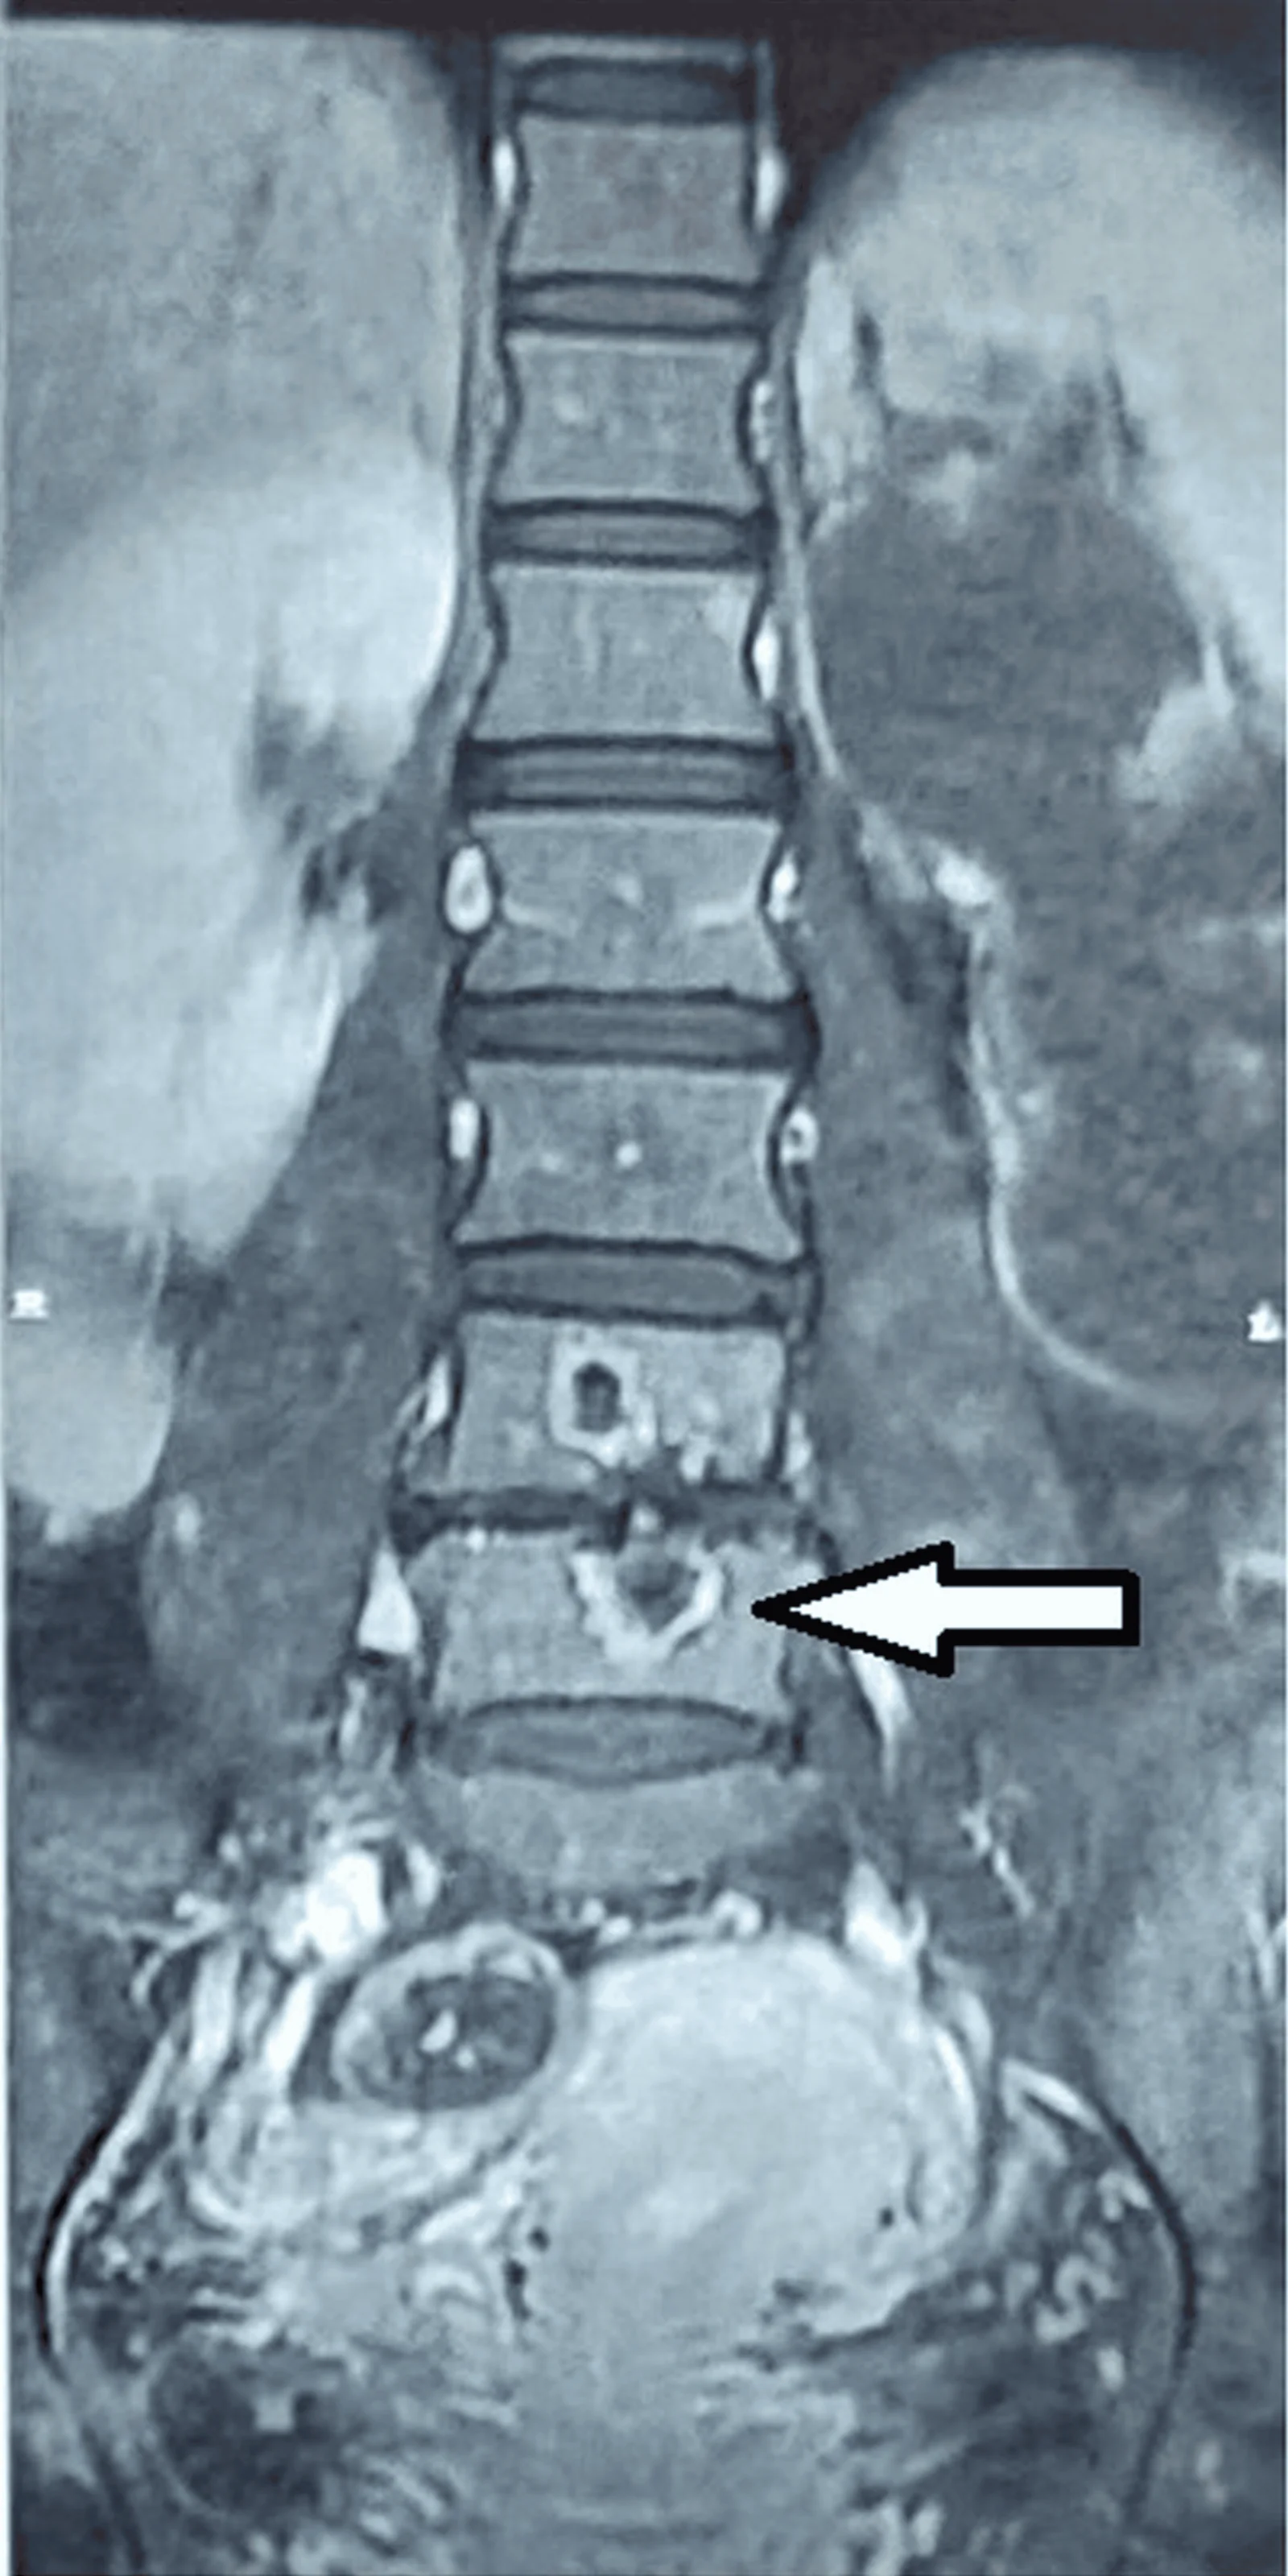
MRI LS spine (coronal view) showing multiple lytic lesions in L4-L5 vertebrae (solid arrow) MRI: magnetic resonance imaging; LS: lumbosacral
